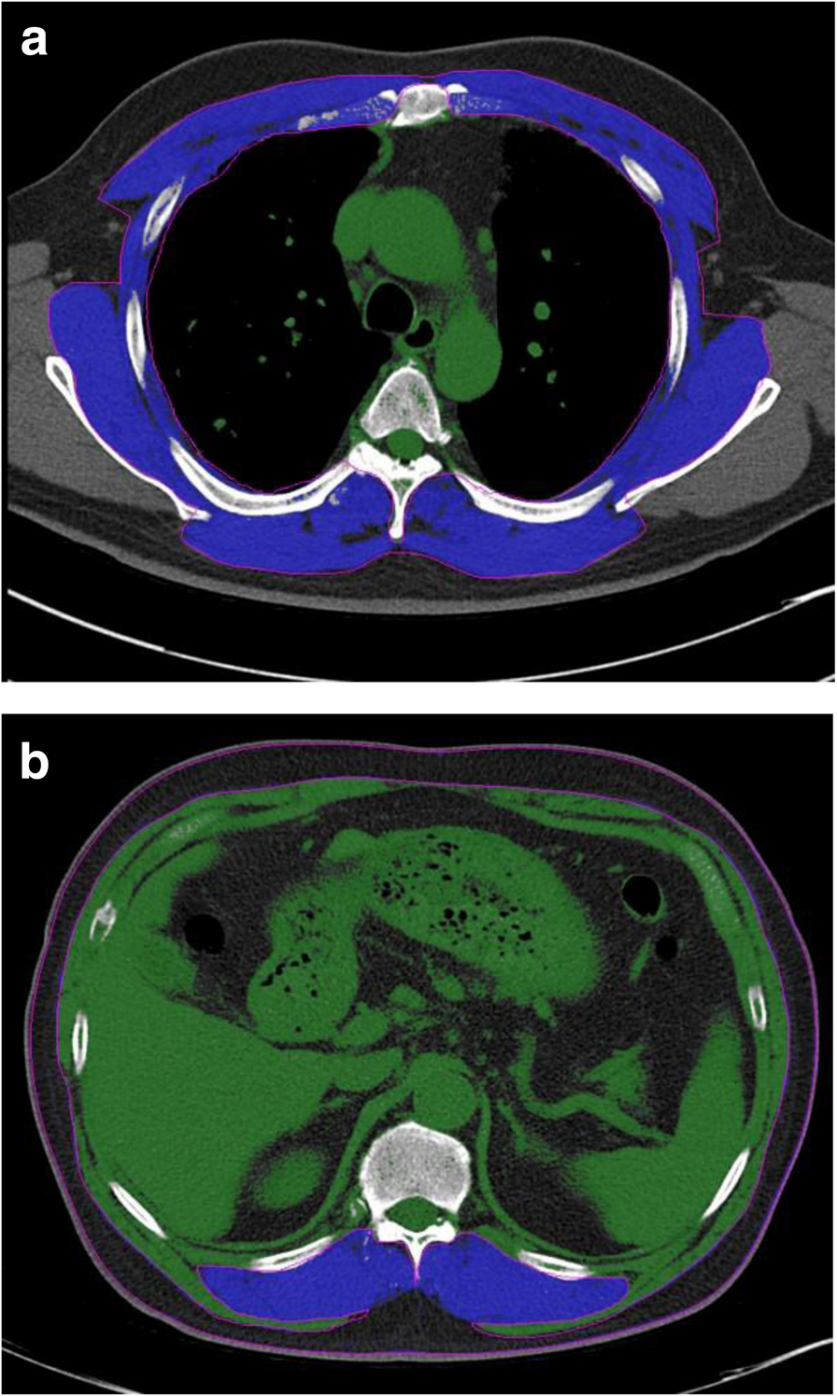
a, b: Sample computed tomography (CT) scans used to determine muscle area in idiopathic pulmonary fibrosis case subjects. Chest CT examinations were obtained by using 24-detector CT scanners (Somatom Emotion 16; Siemens Healthcare, Erlangen, Germany). Non-contrast CTs were used in the analysis. Quantitative assessment of the CSA was performed semi-automatically with Aquarius iNtuition Viewer (ver. 4.4.11, TeraRecon Inc., San Mateo, CA, USA) (a) Axial CT image of the 4th thoracic vertebral region. Pectoralis, intercostalis, paraspinals, serratus, and latissimus muscles are in blue. b Axial CT image of the 12th thoracic vertebral region. Erector spinae muscles are in blue
